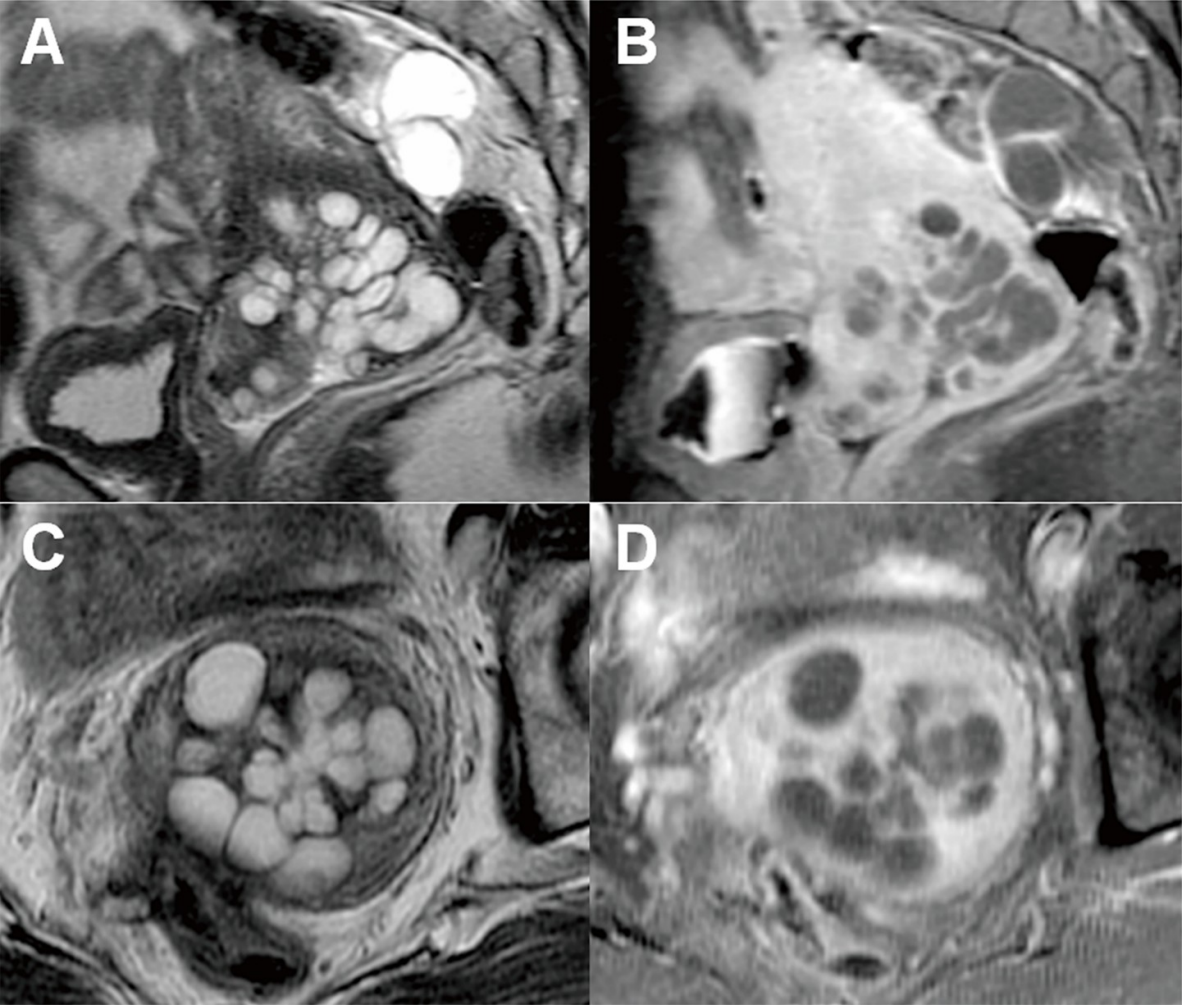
Flower-type multicystic lesion of the cervix. (Case 10) Magnetic resonance images show a lesion of many small cysts surrounded by many larger cysts with hyperintensity on T2-weighted images and hypointensity on contrast-enhanced fat-suppressed T1-weighted images (A and B, sagittal images; C and D, axial images; A and C, T2-weighted imaging; and B and D, contrast-enhanced fat-suppressed T1-weighted imaging).
